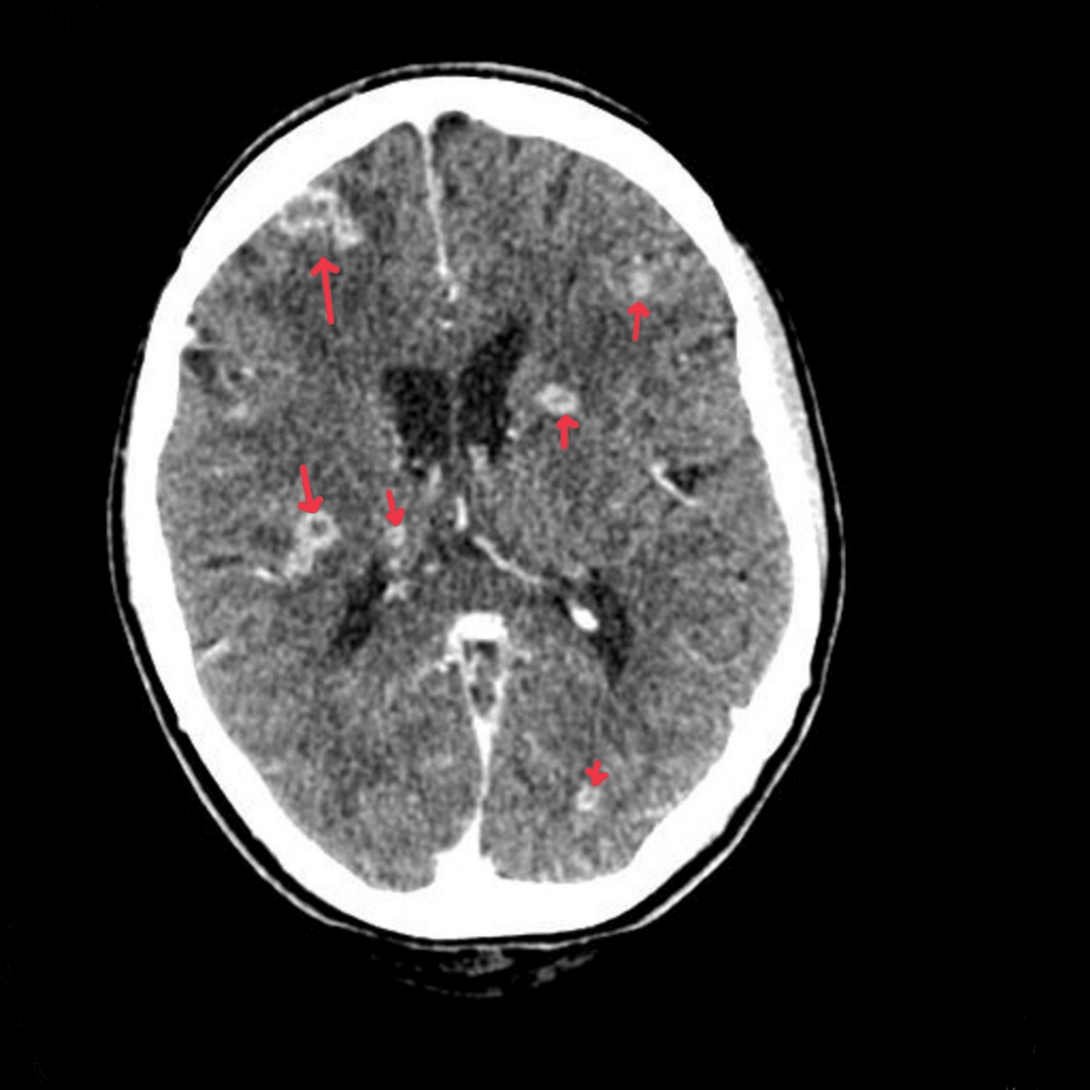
Contrast-enhanced CT head showing multiple ring-enhancing lesions.
